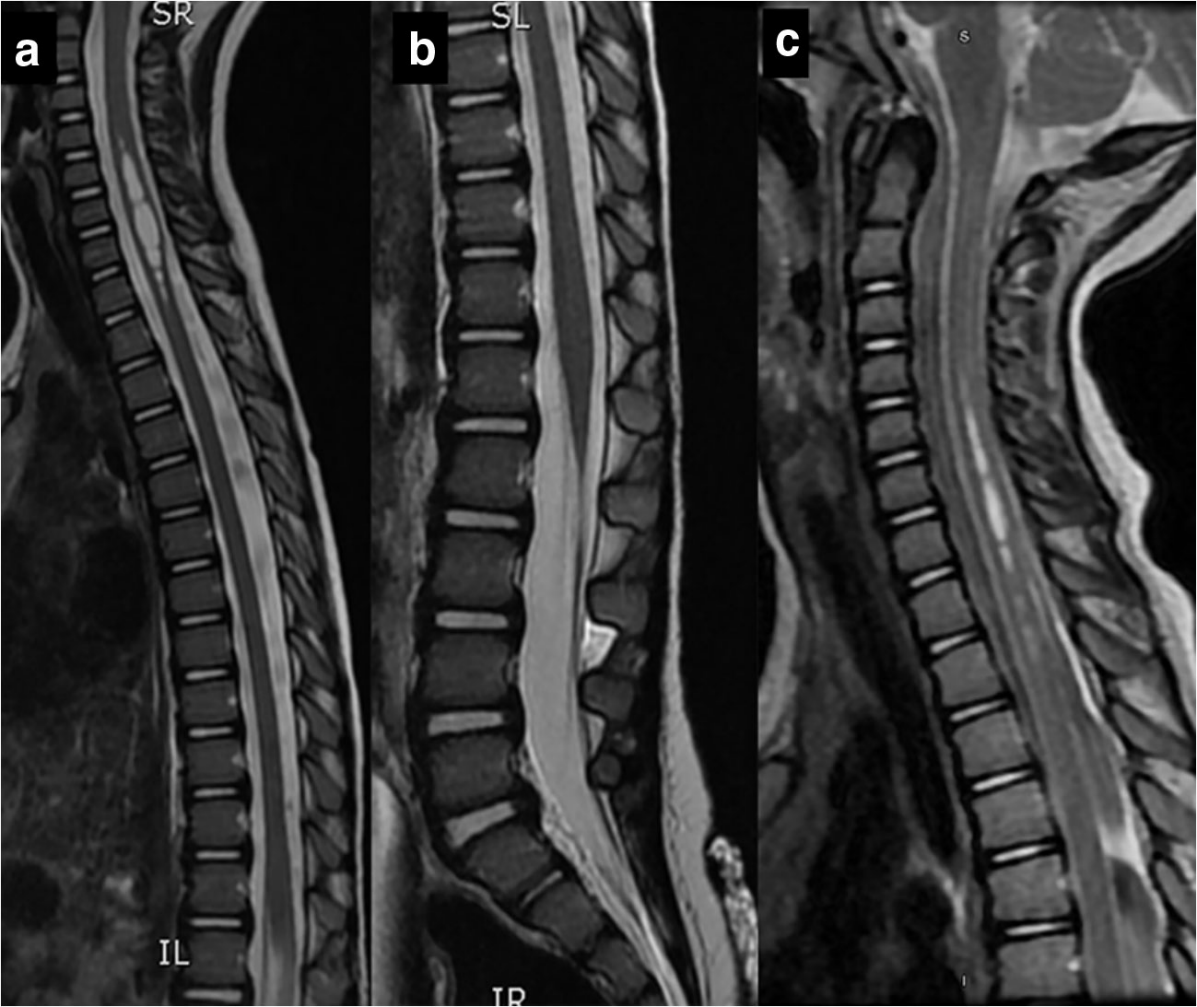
T2 cervicothoracic and lumbar MRI. a Cervicothoracic and b lumbar MRI did not demonstrate any additional lesions or tethering but did reveal cervicothoracic syrinx. c Cervicothoracic MRI demonstrated that the syrinx had diminished in size at two-year follow-up
